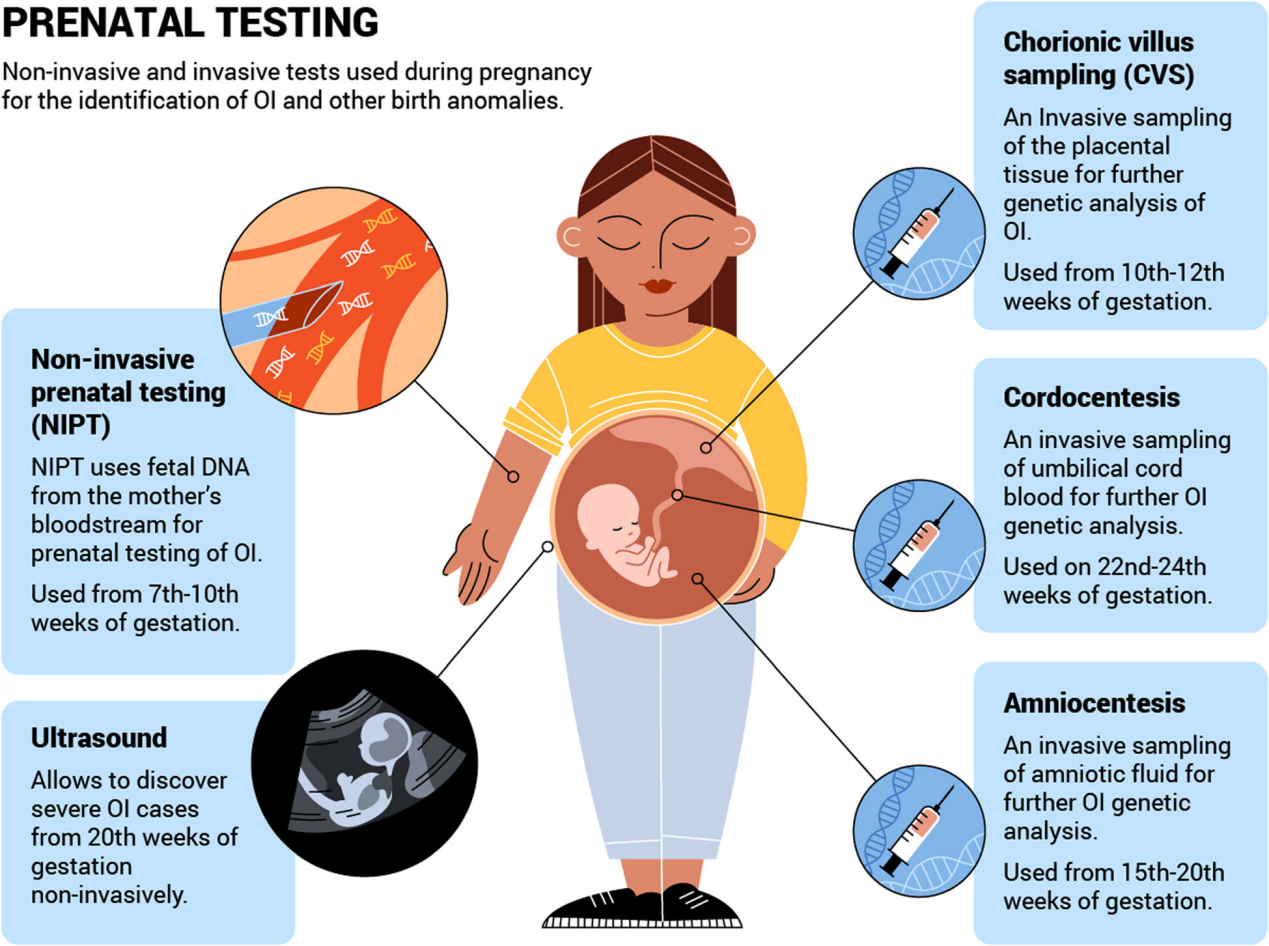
Overview of prenatal testing options for members of families with OI risk. NIPT – non-invasive prenatal testing, ultrasound, CVS - chorionic villus sampling, cordocentesis, amniocentesis
